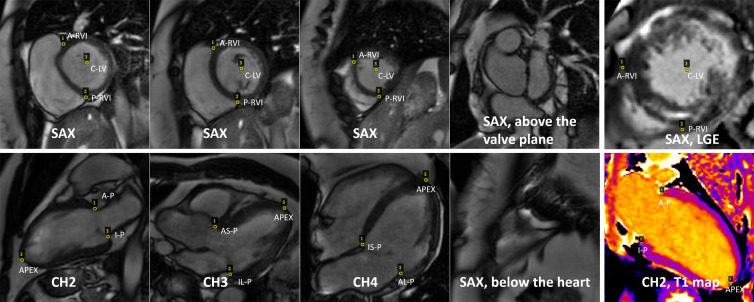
Example of cardiac MR images with landmarks. Three short-axis (SAX) views
